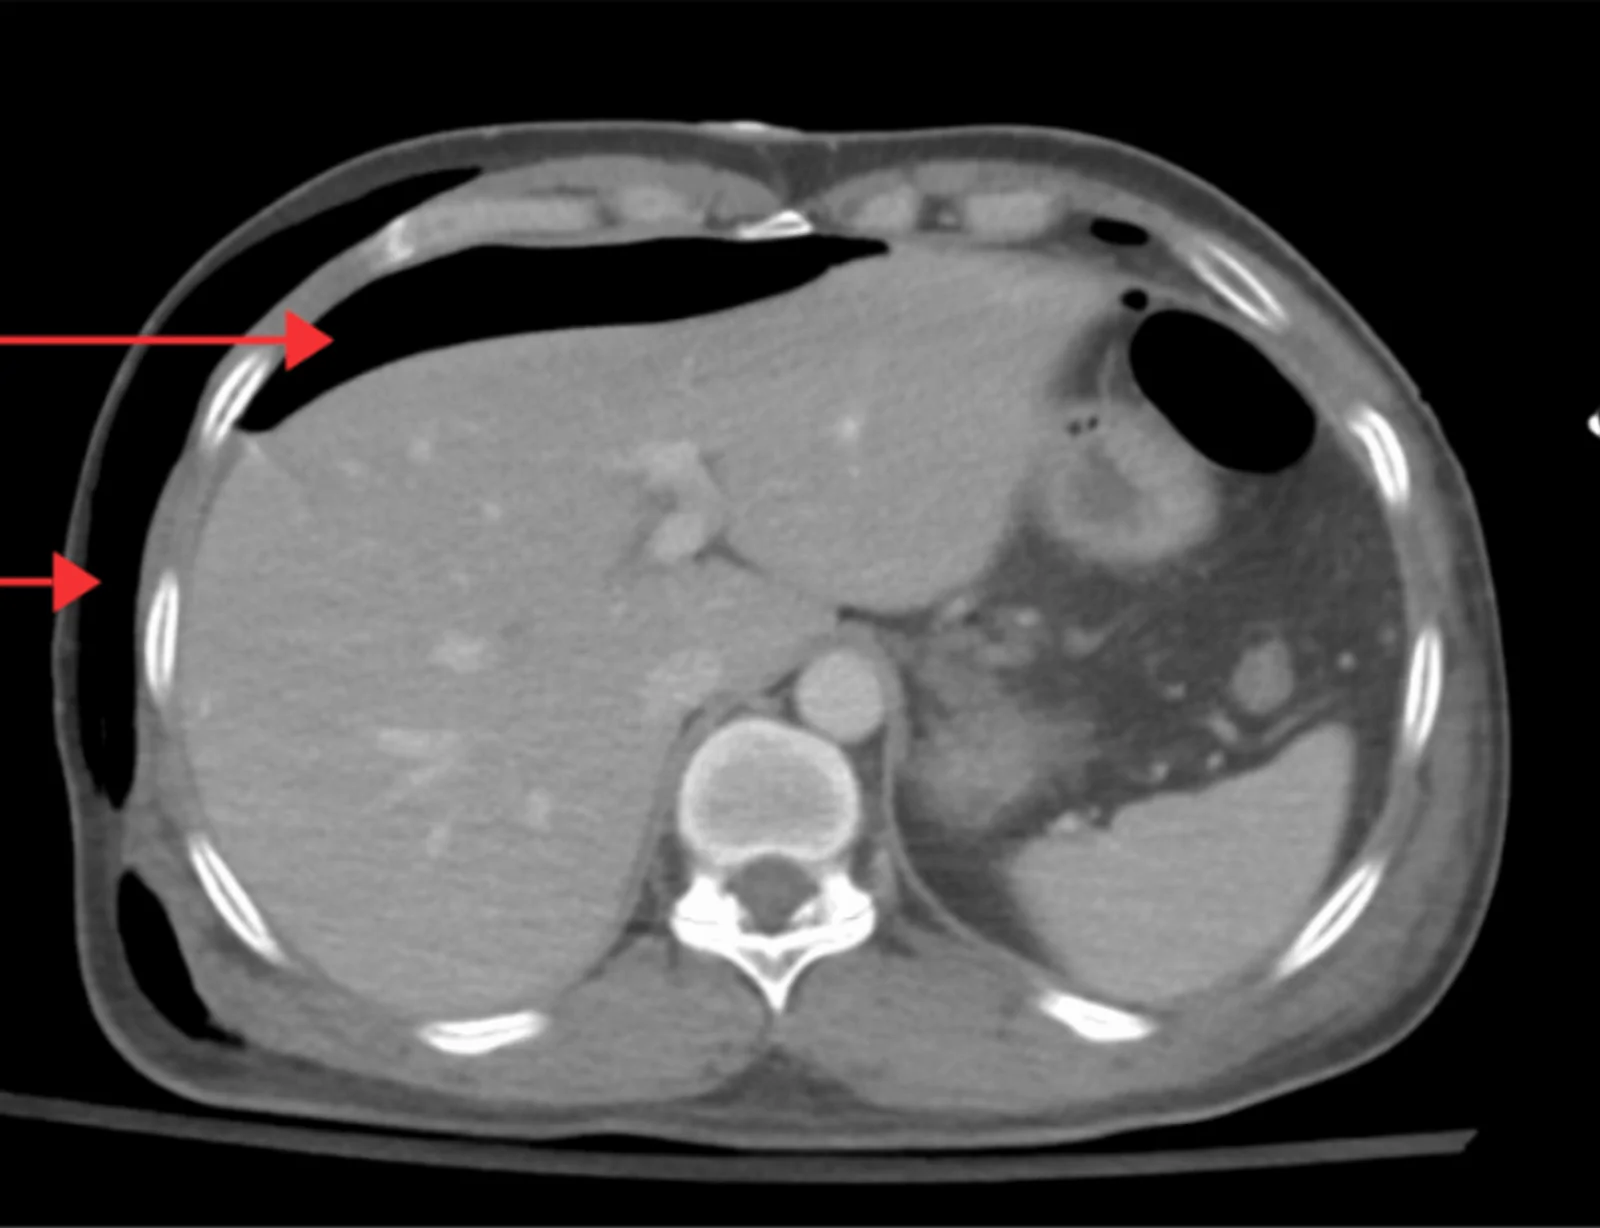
Repeat CT on Day 3 showing significant pneumoperitoneum anterior to the liver with subcutaneous emphysema of the right lateral abdominal wall. The top red arrow points at the significant pneumoperitoneum anterior to the liver The bottom red arrow points at the subcutaneous emphysema of the right lateral abdominal wall.
